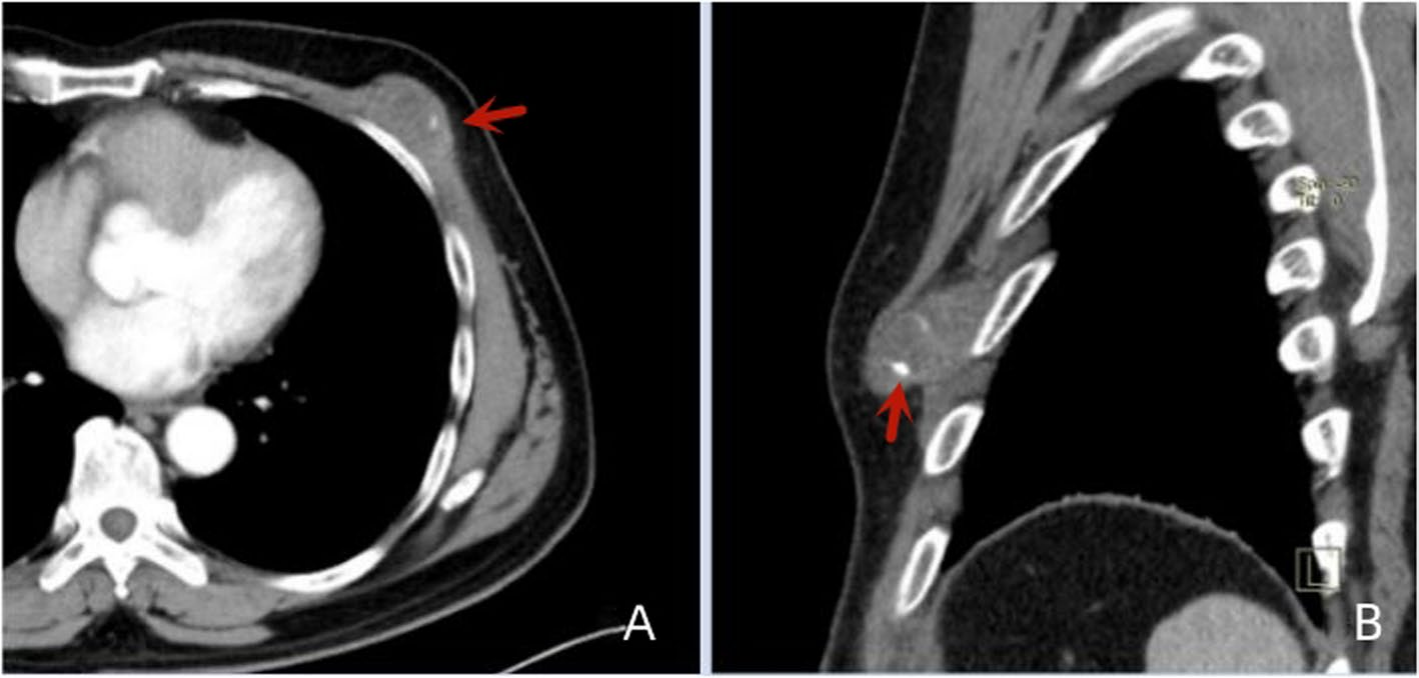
A 62-year-old man with EOS in the left chest wall. A, B Punctate calcifications (red arrows) at the edge of the CT plain scan and mild enhancement of the mass on an enhanced scan
